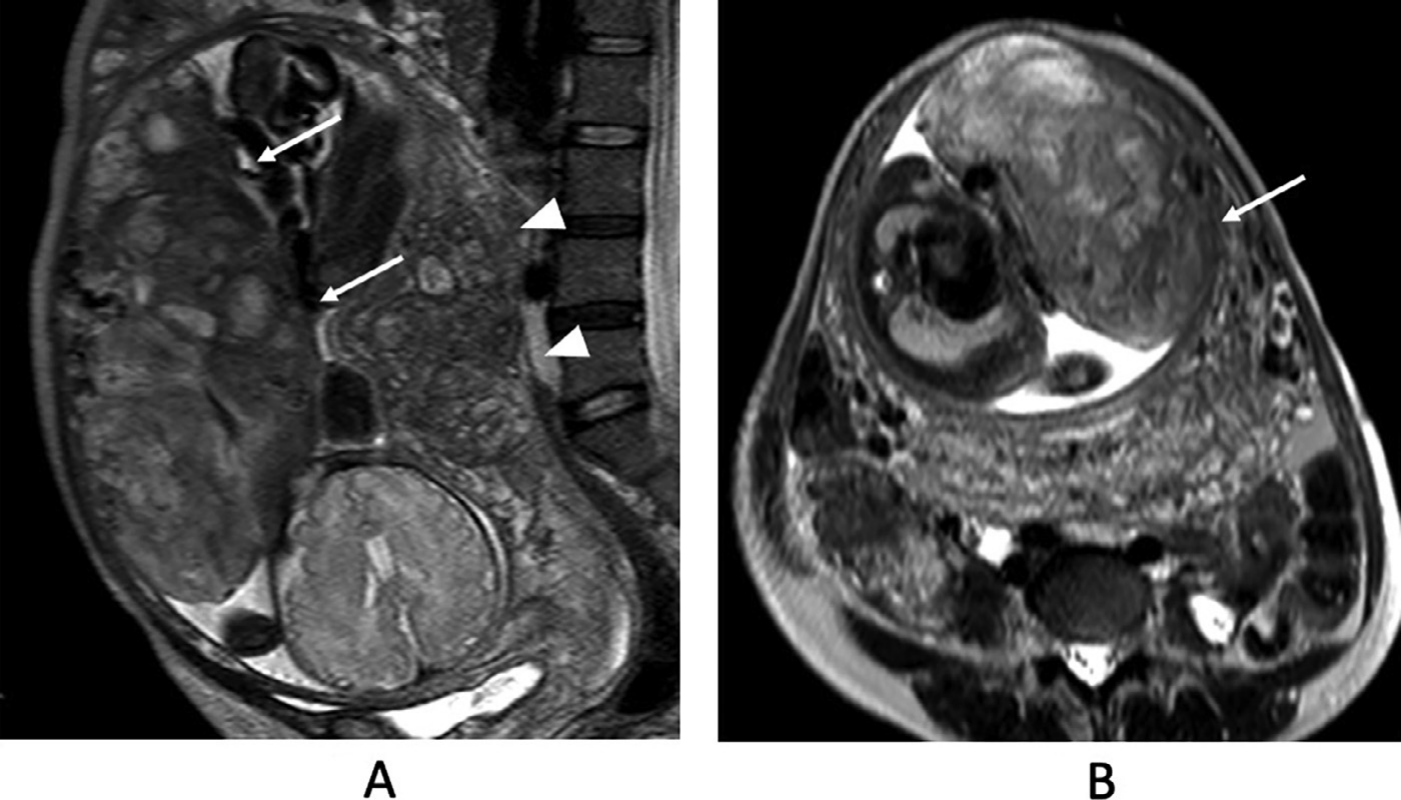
Single-shot turbo spin-echo images (T2-weighted images). (A and B) Magnetic resonance imaging (MRI) obtained at 36 weeks of gestation during the reported pregnancy. T2-weighted images reveal oligohydramnios and a thickened placenta. The placenta shows heterogeneous signal intensity with abnormal intraplacental linear and geographical hypointensity on T2-weighted images (A, B arrows). Adenomyosis is also observed in the posterior uterine wall (A, B arrowheads).
